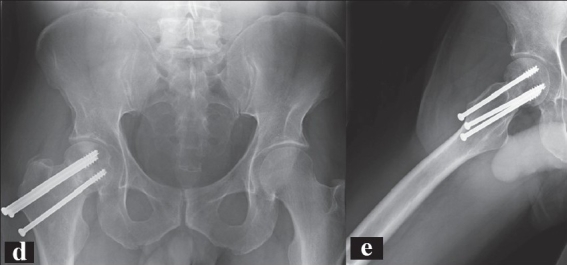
B: AP radiograph (d) and lateral radiograph (e) of the same patient at 8 month followup. There is some settling of the fracture along with the comminution and a lack of complete healing at this point. Functionally, the patient has no pain and is full weight bearing.
